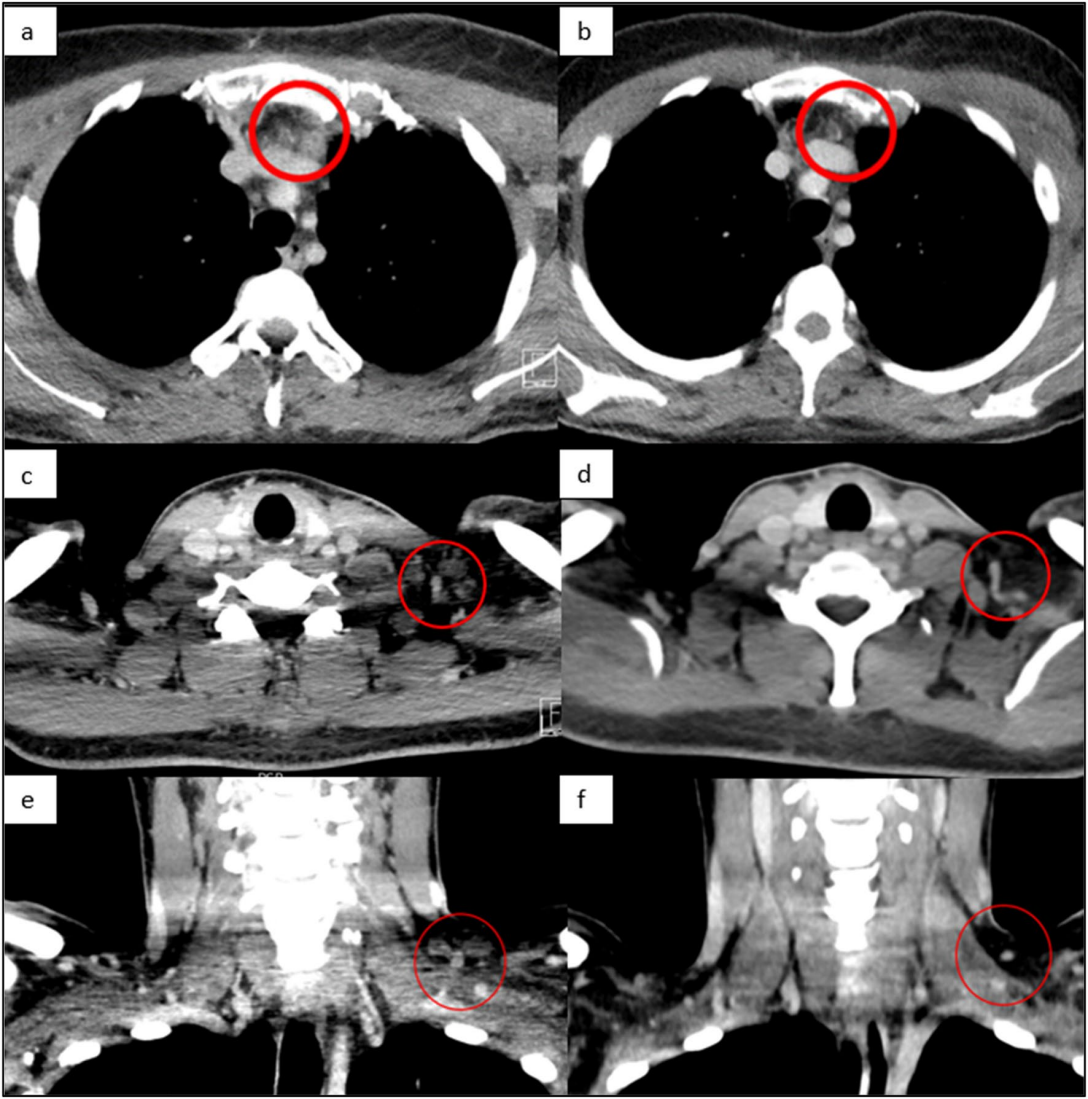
Response to treatment with immunotherapy. a, c, e CT images of the region anterior to the left brachiocephalic vein and the left supraclavicular region in axial and coronal cross sections showing metastatic lymph nodes prior to initiation of therapy with atezolizumab (initially in combination with nab-paclitaxel, which was later discontinued). b After 5 months of treatment, a complete response in the superior mediastinum was seen on CT. d, f After 8 months, there was also a complete response in the supraclavicular region
